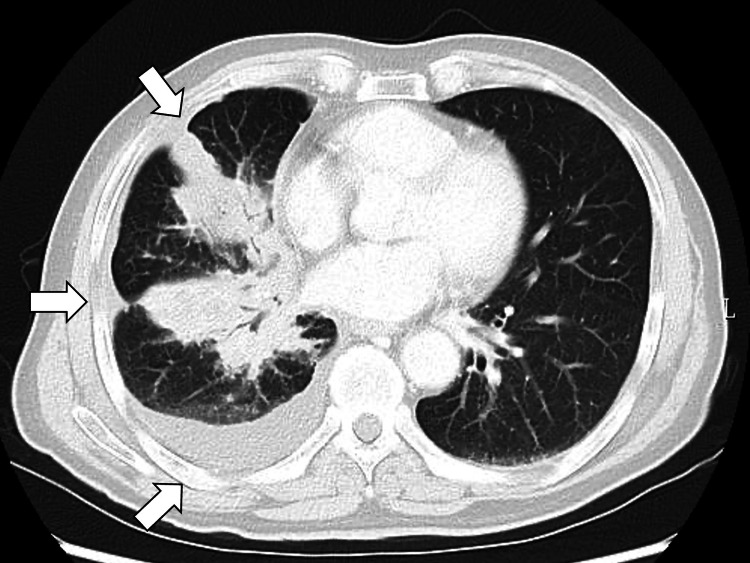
Chest-to-pelvis computed tomography showing a right lower lobe to the hilar mass with pleural thickening and right pleural effusion, suggesting carcinomatous lymphangitis and pleural dissemination (white arrows)
